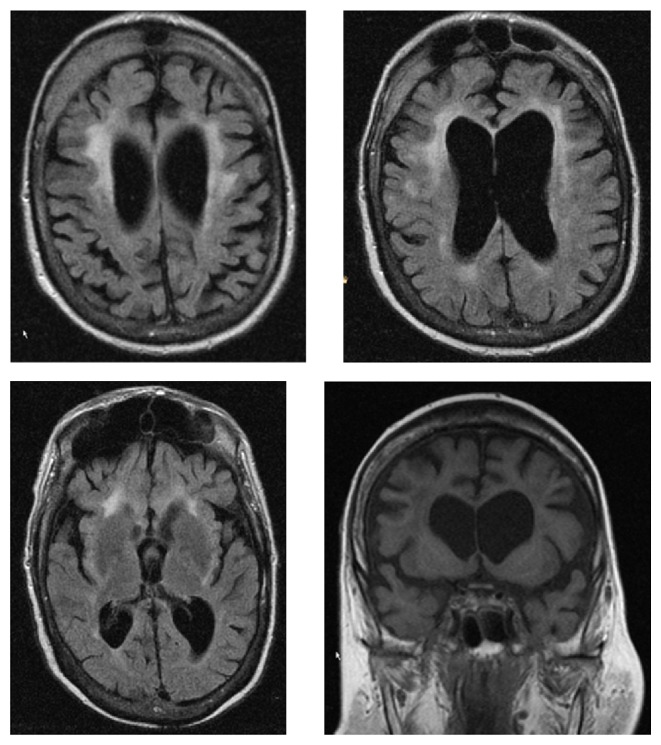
MRI of the cerebrum showing diffuse atrophy gliotic spots and leucoaraiosis. Pneumosinus dilatans can be also seen.
